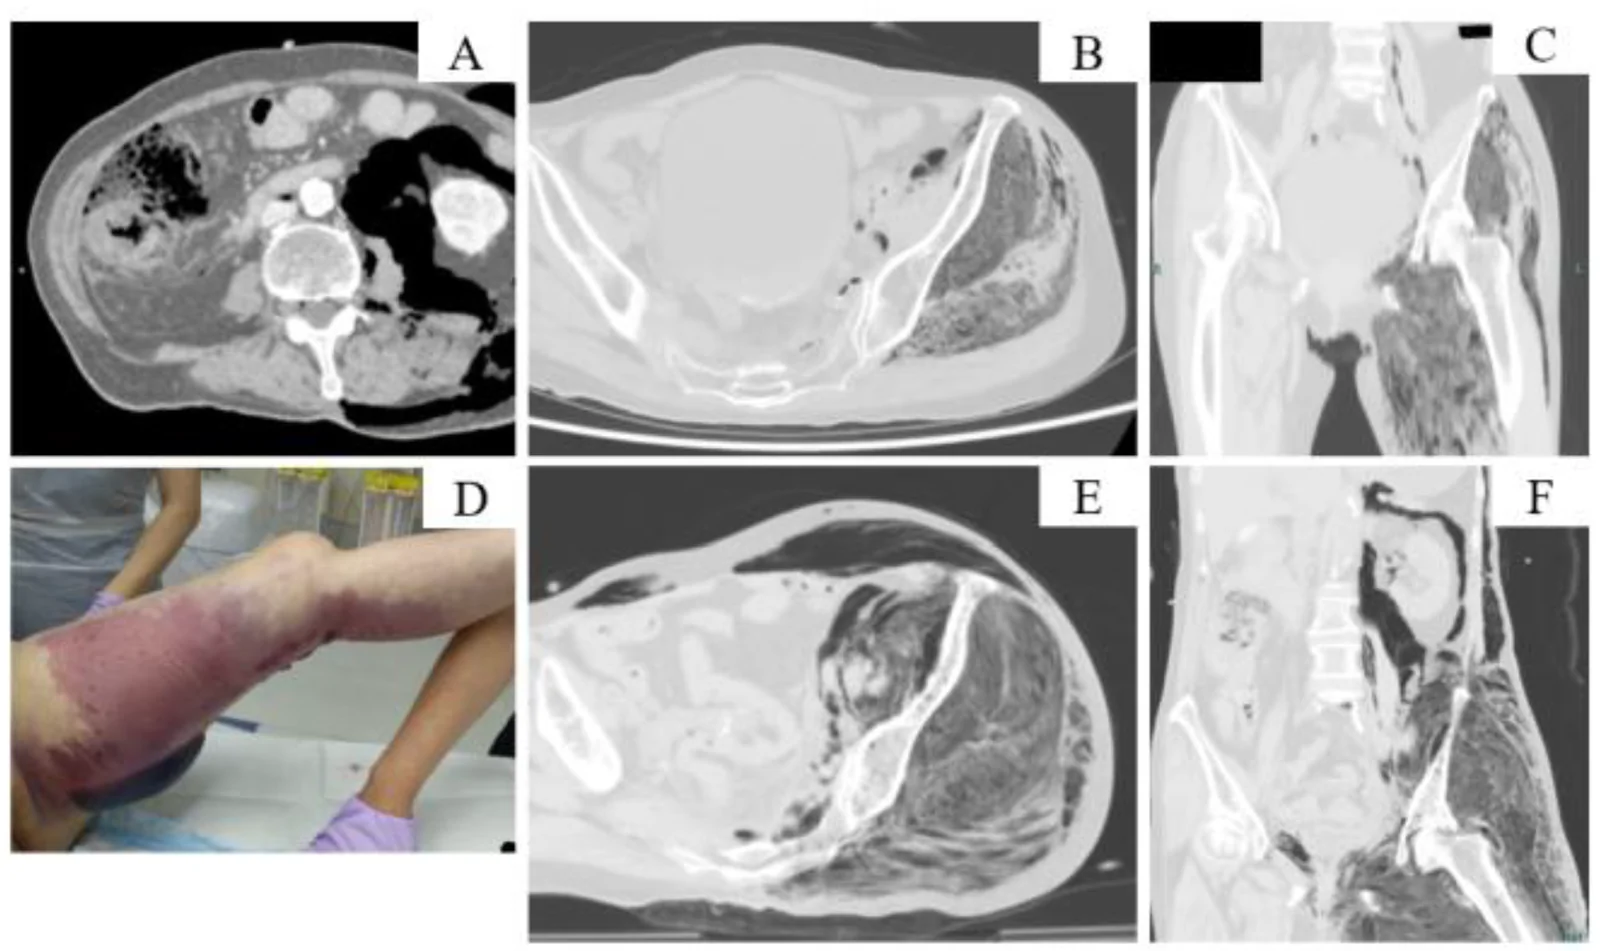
Clinical and radiological findings at presentation. (A) CT performed at the referring hospital shows ascending colon cancer with penetration toward the mesentery. (B, C) CT images obtained at the referring hospital demonstrate extensive gas formation confined to the left thigh and pelvic cavity. (D) Clinical photograph of the left thigh on arrival at our hospital shows marked skin discoloration with a violaceous appearance and bullae formation. (E, F) CT images obtained at our hospital demonstrate rapid progression of gas into the retroperitoneum surrounding the left kidney
